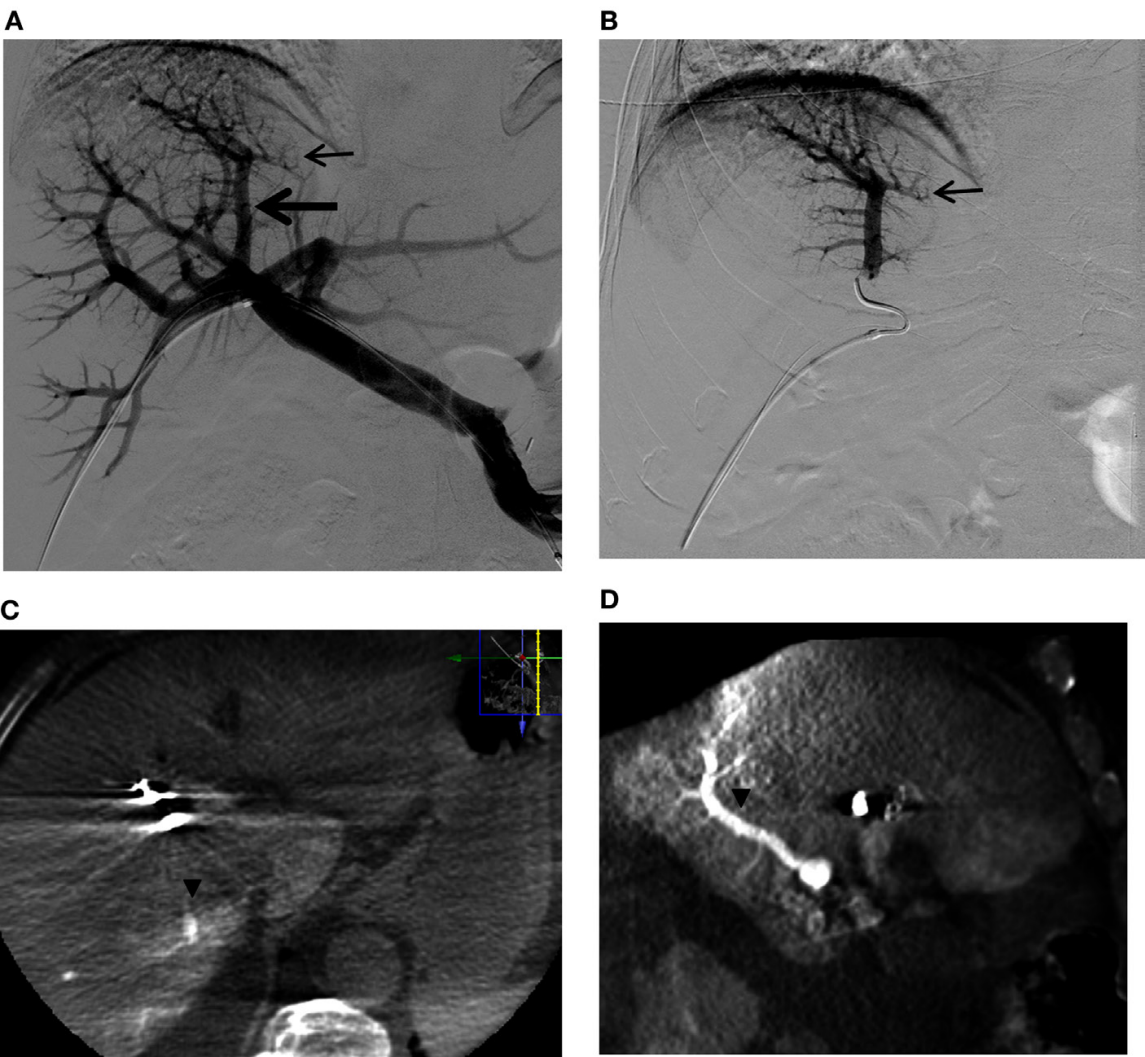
A questionable subsegmental portal vein branch crossing the midplane of the liver in a patient referred for right PVE. (A) Flush portogram shows a subsegmental branch directing to the left (small arrow) that is arising from the segmental branch directing to the dome of the liver (large arrow). (B) Selective right portogram clearly shows the subsegmental branch that possibly supplies segment 4 (small arrow). (C,D) Axial, (A) sagittal, and (B) reformatted C-arm cone-beam CT images during selective right portogram show the segmental branch supplying segment 7 (arrowheads). The subsegmental branch that was considered to be possibly supplying segment 4 was shown to be supplying segment 7. This segmental branch was then confidently embolized with PVA particles and coils. Note that artifact from the metallic coils placed in the right liver during the PVE procedure.
